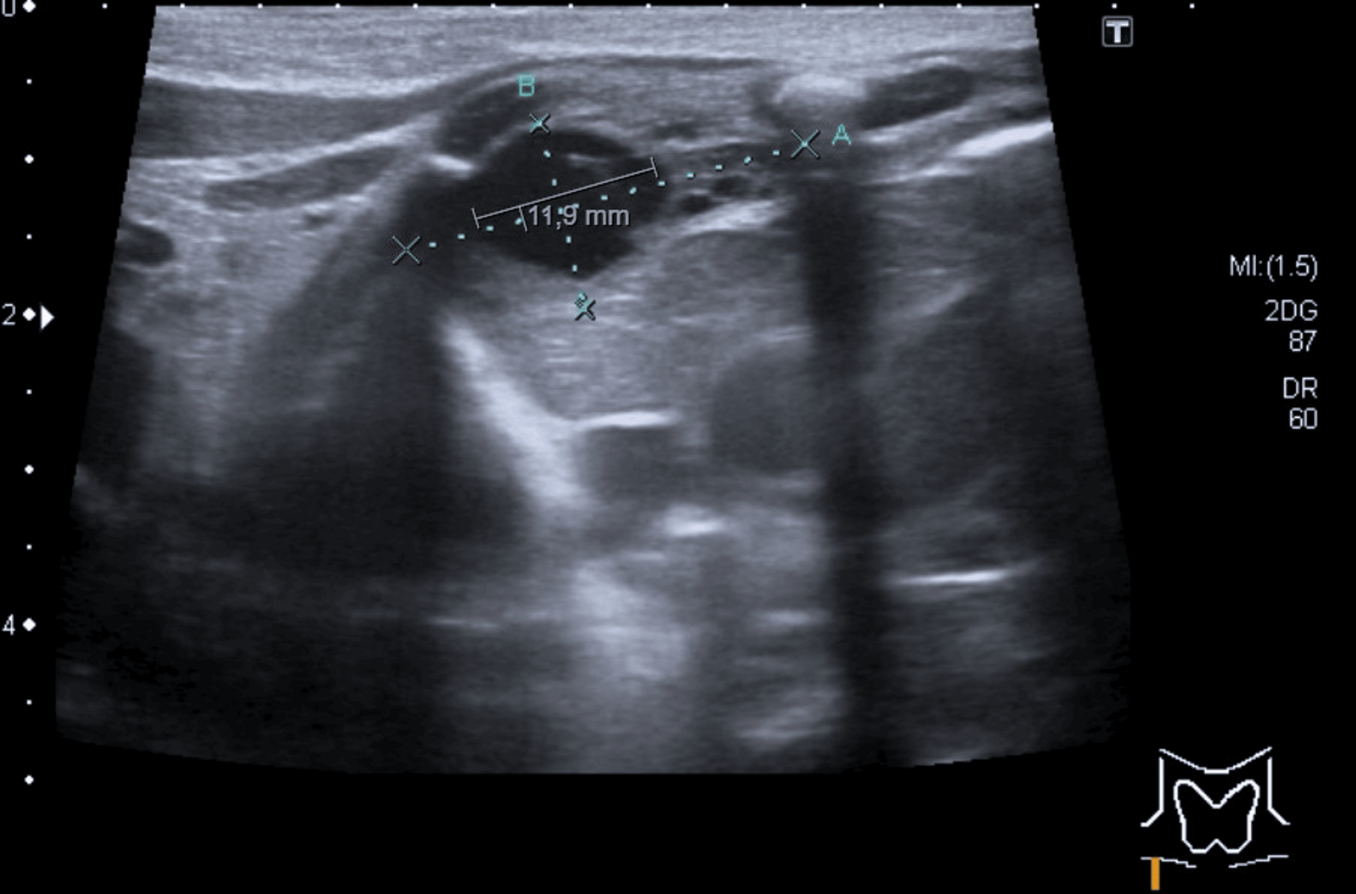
Lymphangioma in the anterior mediastinum Ultrasound image of the anterior mediastinum showing a right-lateralized, multiloculated cystic lesion (measuring 31 × 27 × 12 mm), located anterior to the thymus. The lesion is avascular on Doppler evaluation and is suggestive of a lymphangioma (axial view).
